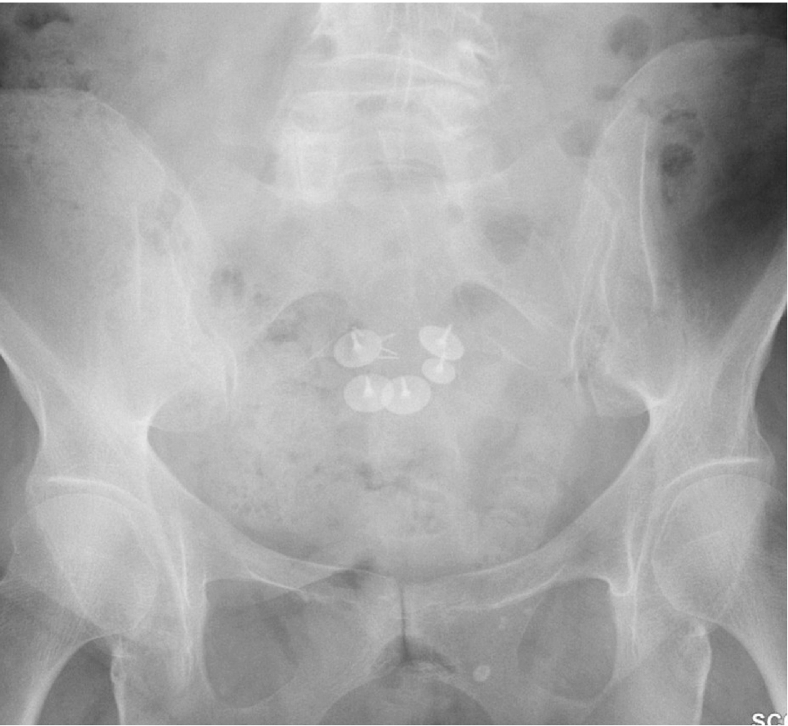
Post-operative X-ray of Hemorrhage Occluder™ Pin placement. Post-operative kidney, ureter, bladder (KUB) X-ray showing location of Hemorrhage Occluder™ Pins.
